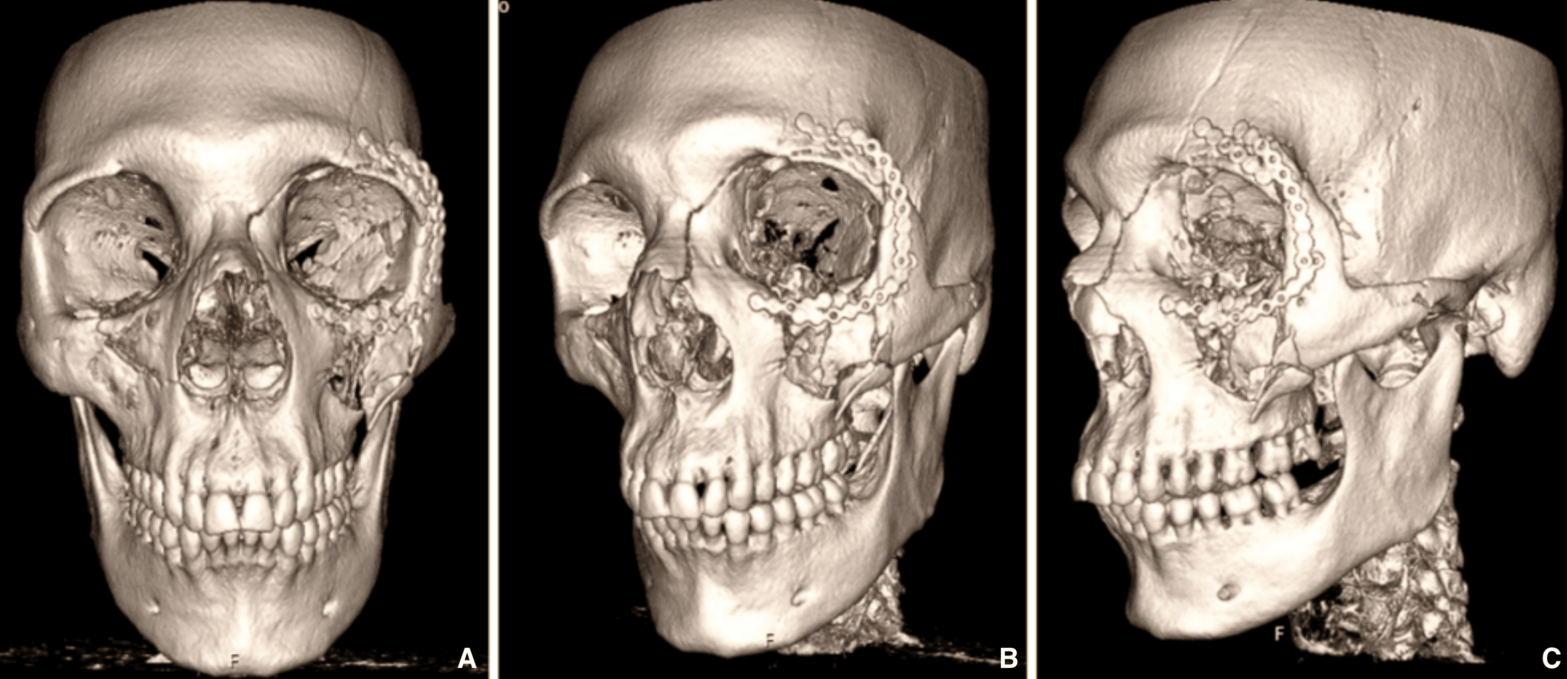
Postoperative CT imaging - 3D reconstruction. The first image (A) corresponds to the anterior-posterior view; the second image (B) corresponds to the left oblique view; and the third image (C) corresponds to the left lateral view.
